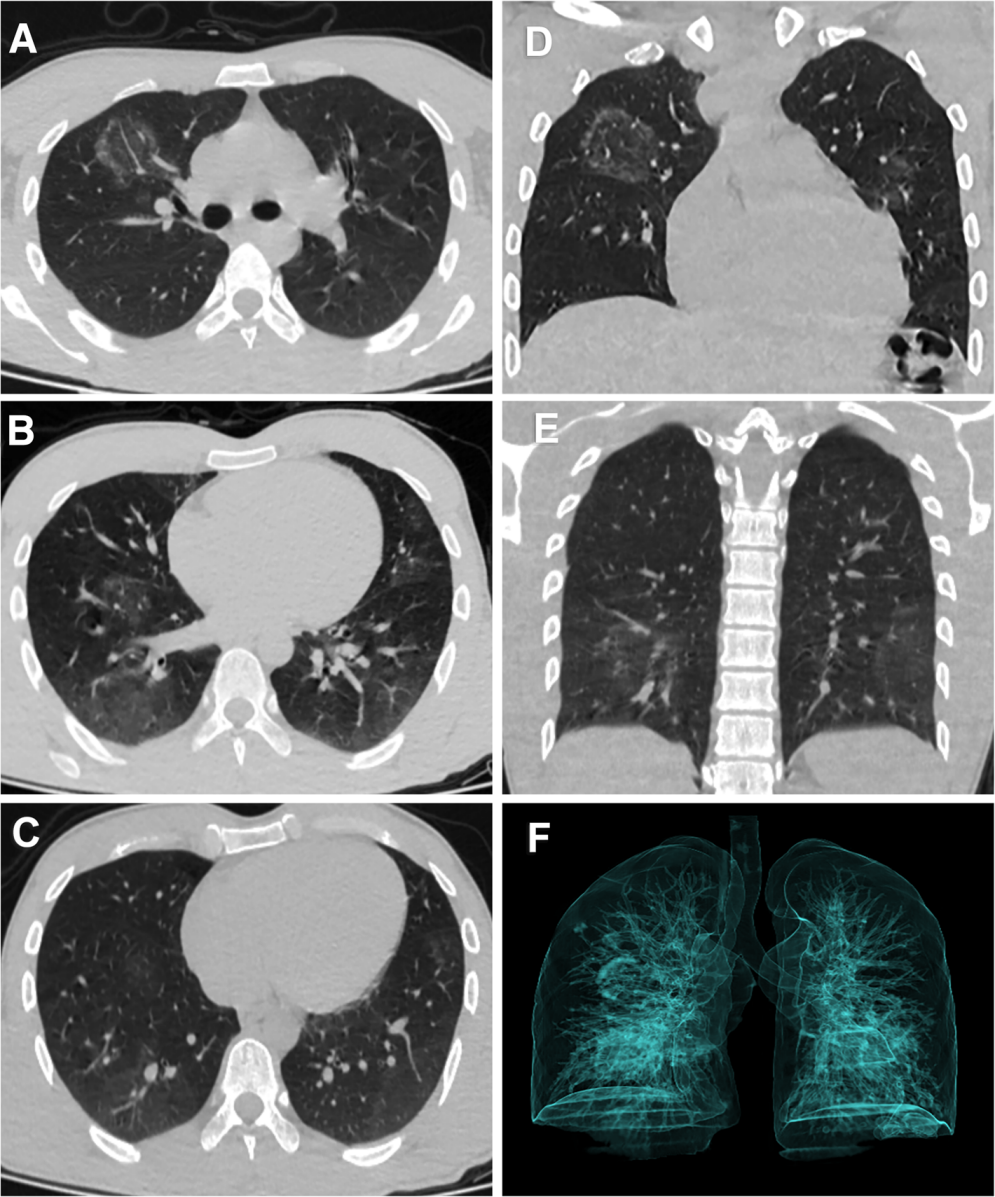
Non-contrast axial CT of the chest of a 30-year-old male patient presented with fever and dyspnea with positive RT-PCR. The right upper lobe shows a reverse halo “atoll sign” in axial and coronal cuts (a, d). Bilateral ground-glass opacities are seen at lower lobes with peripheral distribution (b, c, e). 3D reconstruction volume rendering image shows pulmonary involvement as opaque non-transparent shadows (f). CT severity score is 7 points which corresponds to mild involvement. The patient improved on follow-up
